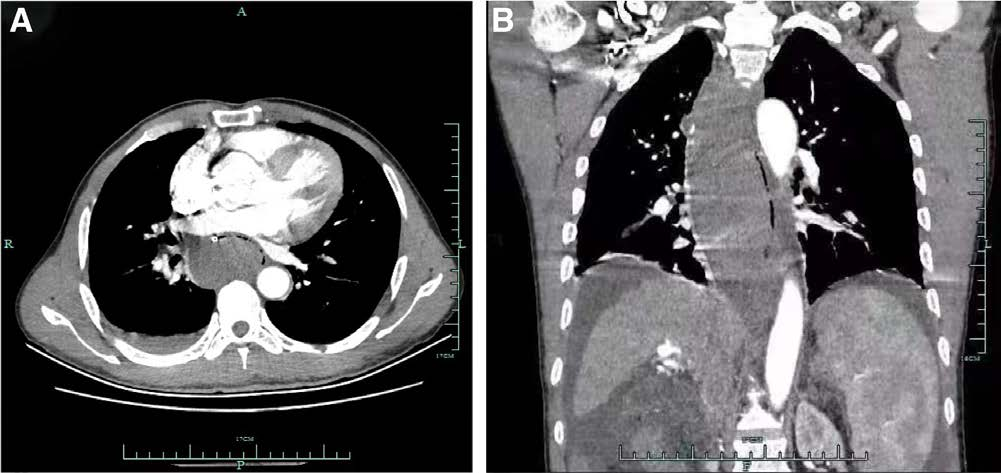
Chest CT images. (A) Axial view showing esophageal stenosis and a soft tissue opacity. (B) Nonenhanced esophageal mass involving the entire esophagus in the mediastinum. CT = computed tomography.
